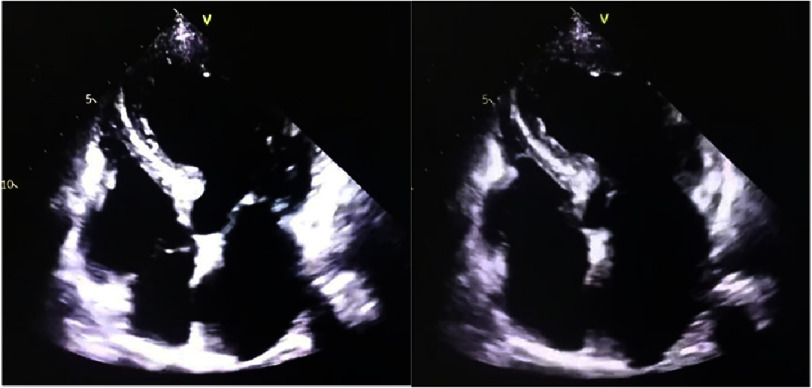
Apical four-chamber echocardiography showing the ballooning aspect of the left ventricle at admission.
